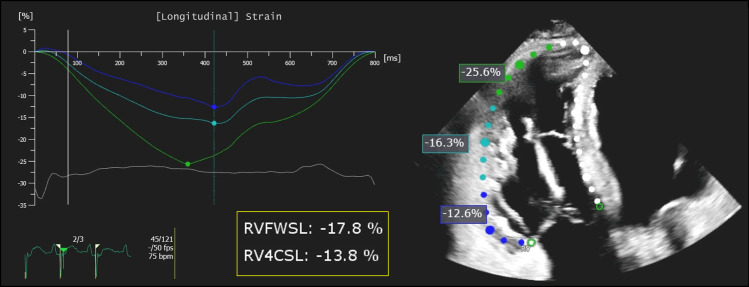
Assessment of right ventricular longitudinal strain using speckle-tracking echocardiography from an apical four-chamber view. The curves display longitudinal strain values over time for individual free-wall RV segments (basal, mid, apical). RVFWSL, right ventricular free wall strain; RV4CSL, right ventricular four-chamber longitudinal strain.
